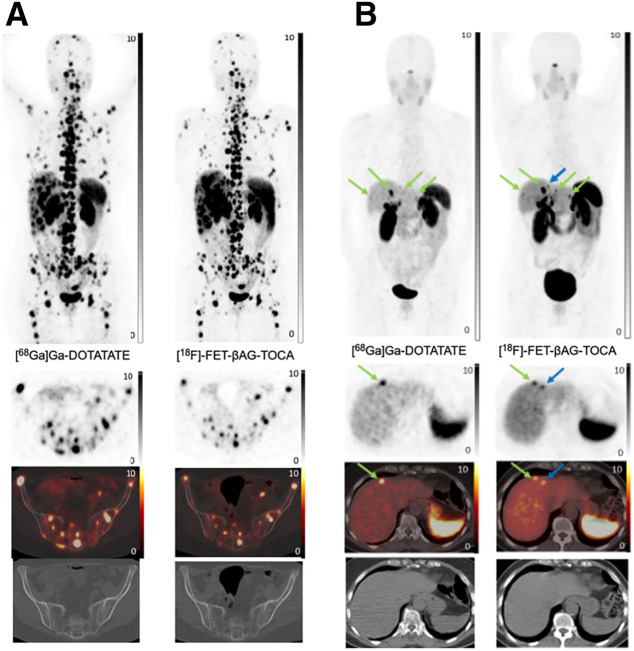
(A) Congruent imaging: [^68^Ga]Ga-DOTATATE imaging and [^18^F]FET-βAG-TOCA imaging (maximum-intensity projection [MIP], axial PET, fused and CT images) in metastatic small-bowel NEN with widespread liver and bone metastases. (B) Incongruent imaging: [^68^Ga]Ga-DOTATATE imaging and [^18^F]FET-βAG-TOCA imaging (MIP, axial PET, fused and CT images) performed 4 wk apart in metastatic ileal NEN with liver metastases (green arrows), which are more visible on [^18^F]FET-βAG-TOCA. Additional lesion is detected on [^18^F]FET-βAG-TOCA (blue arrow).
